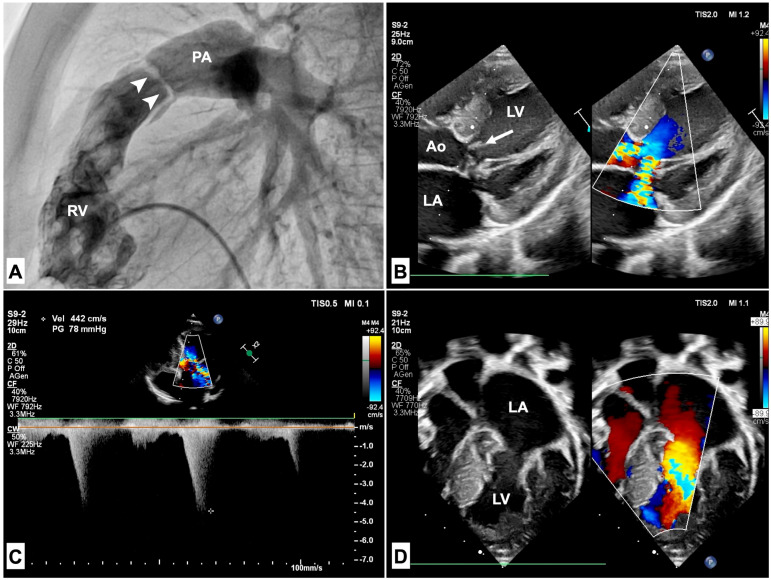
(A): RV angiography shows a pulmonary valve (arrows) with thickened leaflets, doming systolic motion, and tight orifice, causing severe pulmonary valve stenosis. The PA trunk is not dilated, as the valve is characterized mostly by dysplasia rather than a fusion of commissures. (B,C): Echocardiographic findings of HCM in an NSML patient. The ventricular septum is sigmoid with a septal bulge. During systole, anterior systolic motion of the anterior mitral leaflet (arrow) causes severe LVOTO and mitral regurgitation, with a peak gradient of nearly 80 mmHg (C). (D): In an NS patient with HCM, severe LV hypertrophy is associated with a biconvex shape of the ventricular septum. Anomalous insertion of mitral chordae is also displayed. Ao: aorta; LA: left atrium; LV: left ventricle; PA: pulmonary artery; RV: right ventricle.
